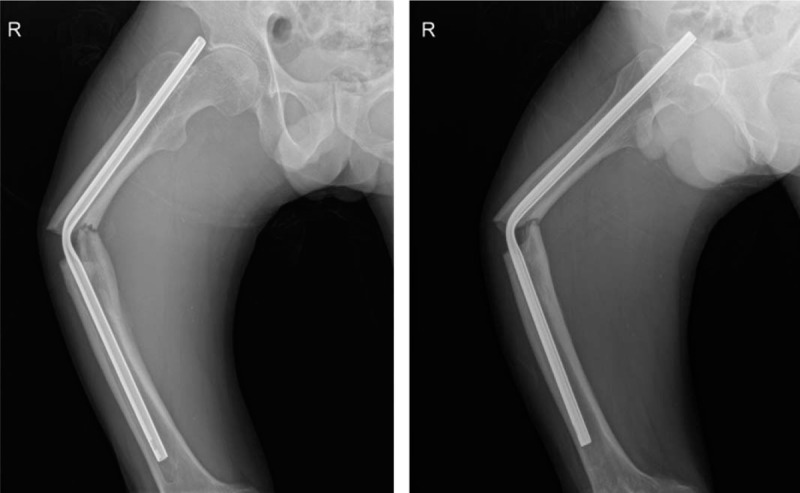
Radiographs showing a refracture of the right femoral shaft and a bent IM nail with 60.1° varus deformity.
